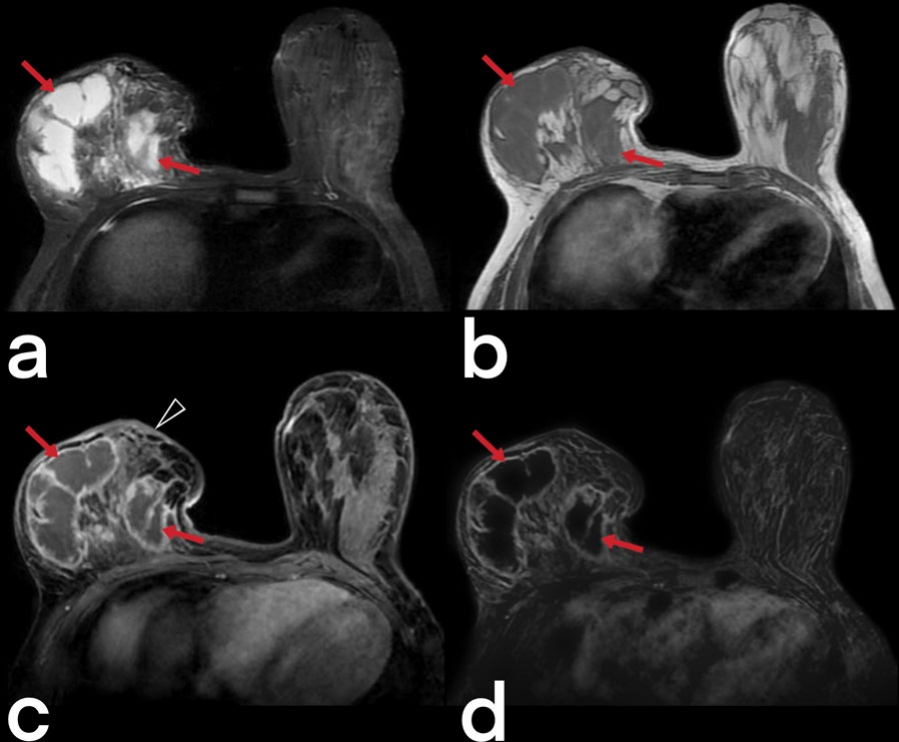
Breast MR images of a 39-year-old female. T2 weighted image (a), T1 weighted image (b) post-contrast (c), and subtracted image (d) demonstrate thickening of the skin (arrowhead) and multiple abscesses (arrows) with peripheral enhancement in the right breast
